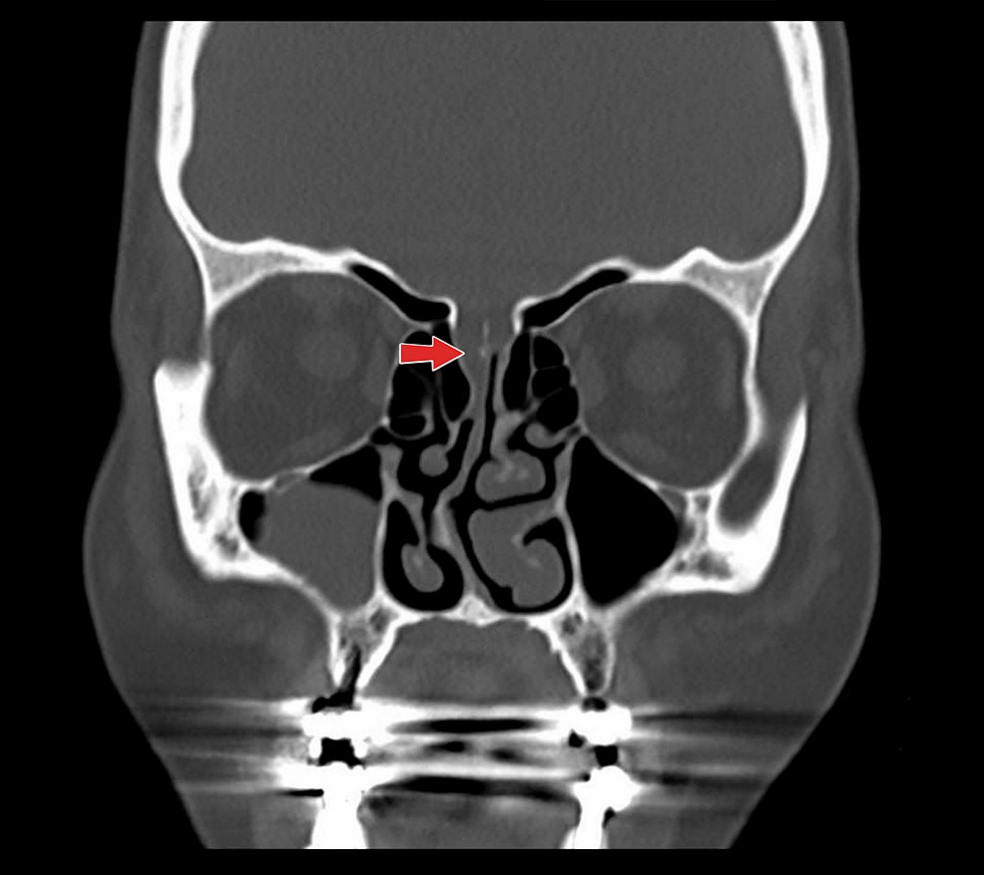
Computed Tomography (CT) sinuses showing a probable defect seen in the right side of the cribriform plate (red arrow), with fluid density seen at the upper nasal cavity.
